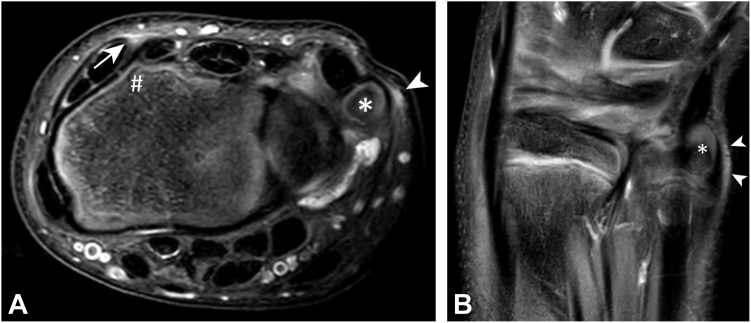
T2-weighted fat saturated MRI of the same asymptomatic gymnast with high signal intensity within subcutaneous fat on the dorsal wrist (arrow) surrounding lister’s tubercle (hashtag) of the distal radius and on the ulnar side (arrow head) of the styloid process (asterisks) suggesting a sort of dorsal as well as ulnar wrist impingement. A Axial T2-weighted fat saturated MRI. B Coronal T2-weighted fat saturated MRI.
